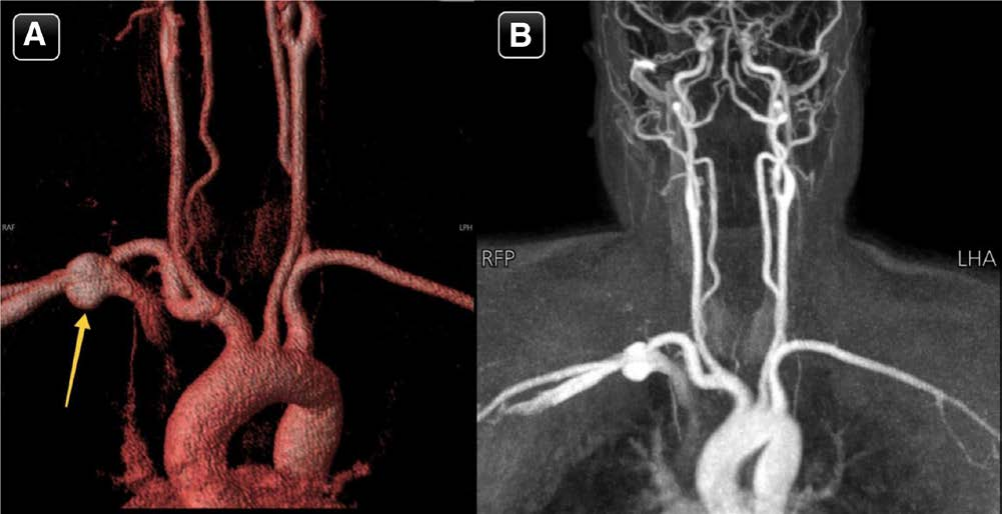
(A & B) Sagittal and axial sections of T2WI cervical spine MRI showing mild diffuse disc bulge more to the left side (red arrows) causing mild central canal and left neural foraminal stenosis. magnetic resonance imaging
